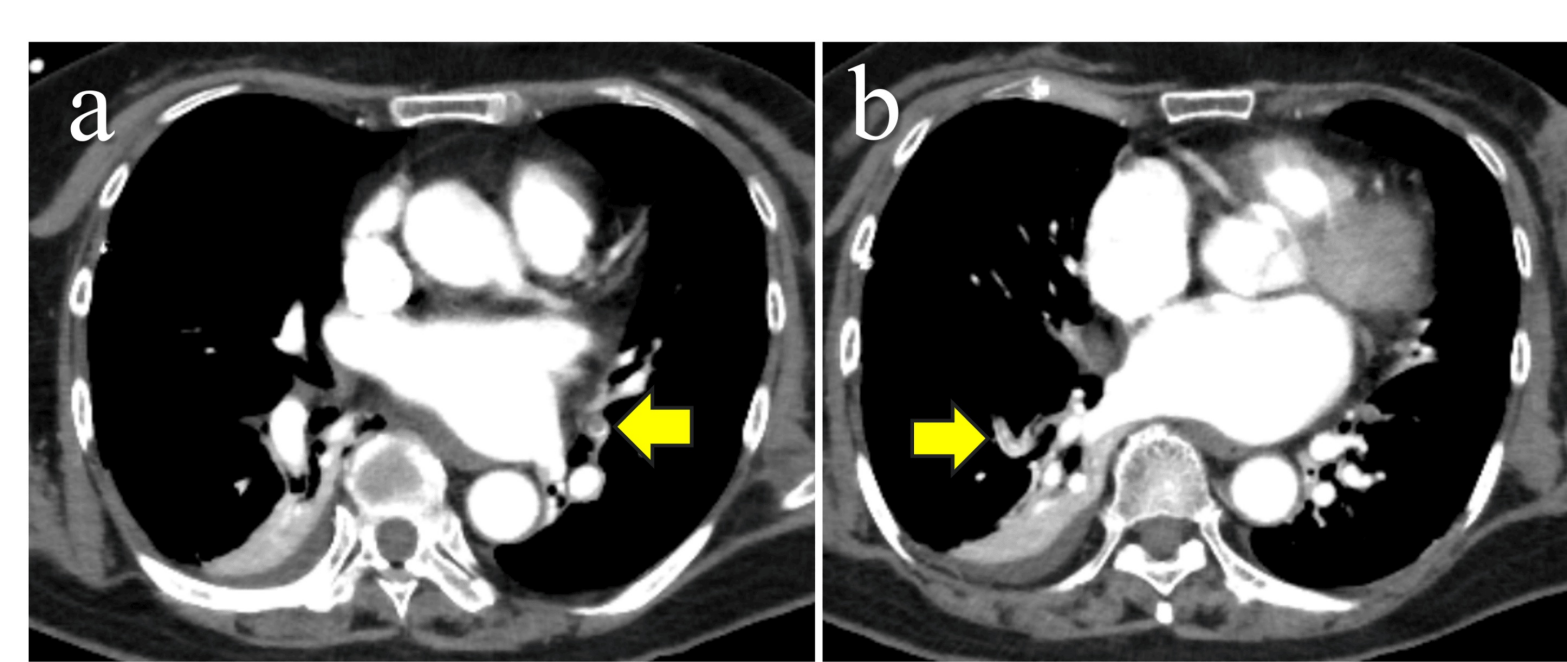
Contrast-enhanced CT findings of pulmonary embolism Multiple emboli in the pulmonary arteries of the right lung (a) and left lung (b) (arrows).
